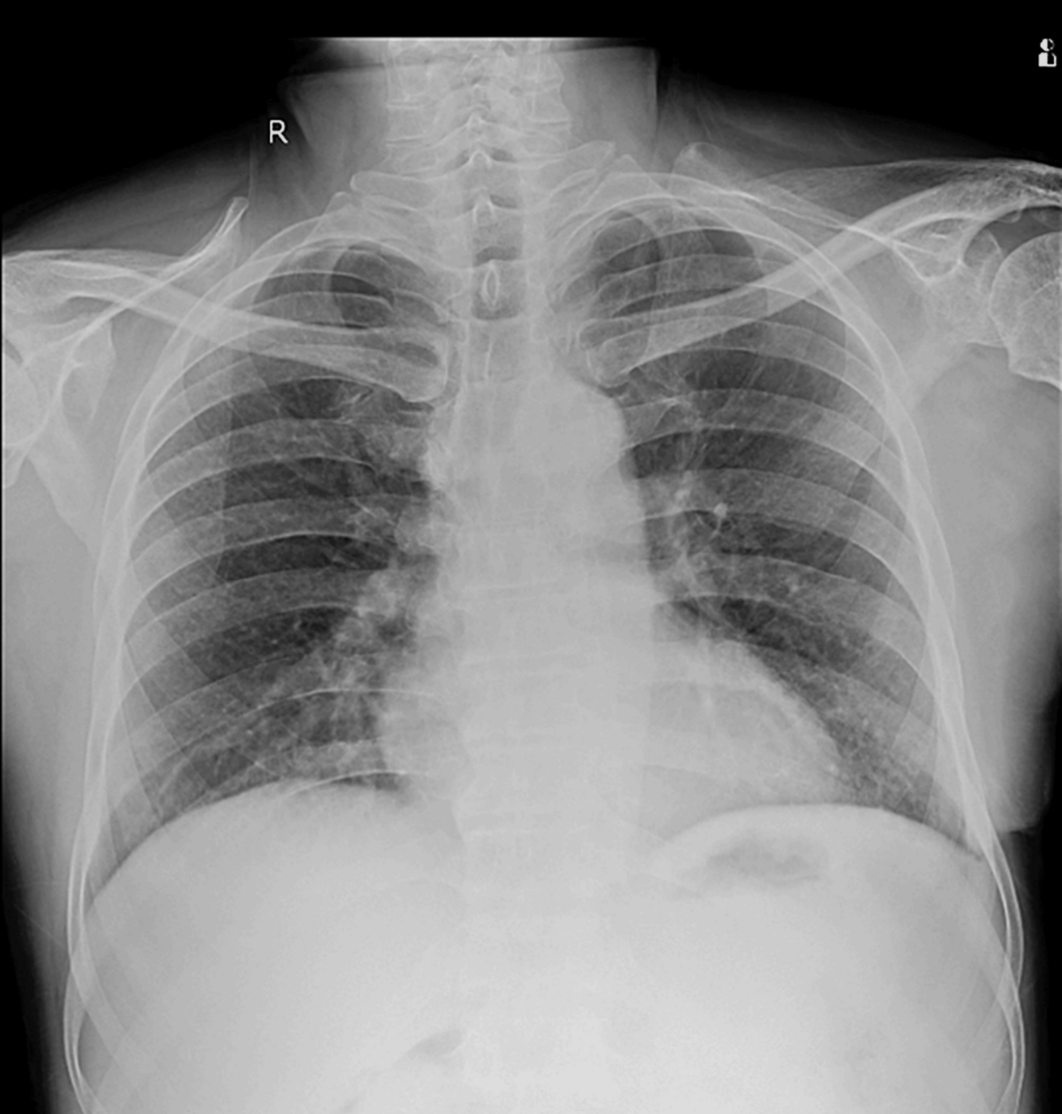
Chest X-ray six months post-operative showing complete resolution
